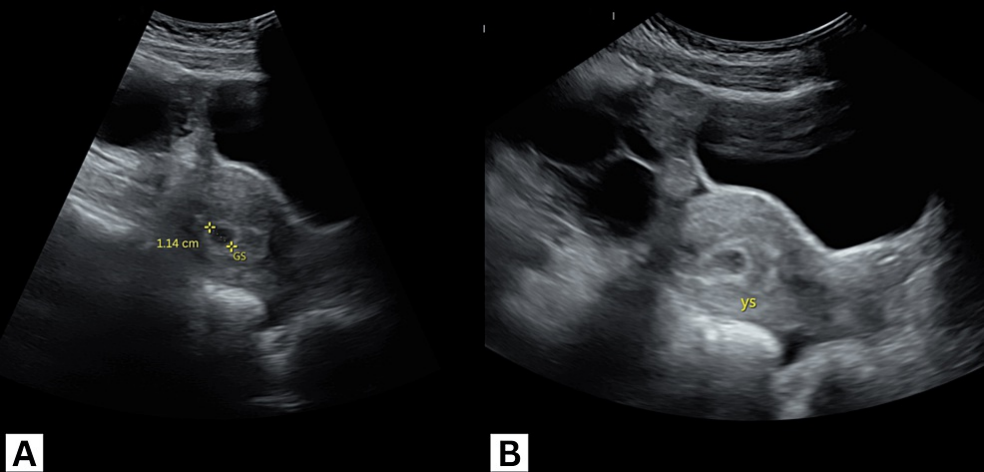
Transabdominal ultrasound images showing (A) gestational sac (GS) and (B) yolk sac (YS).
